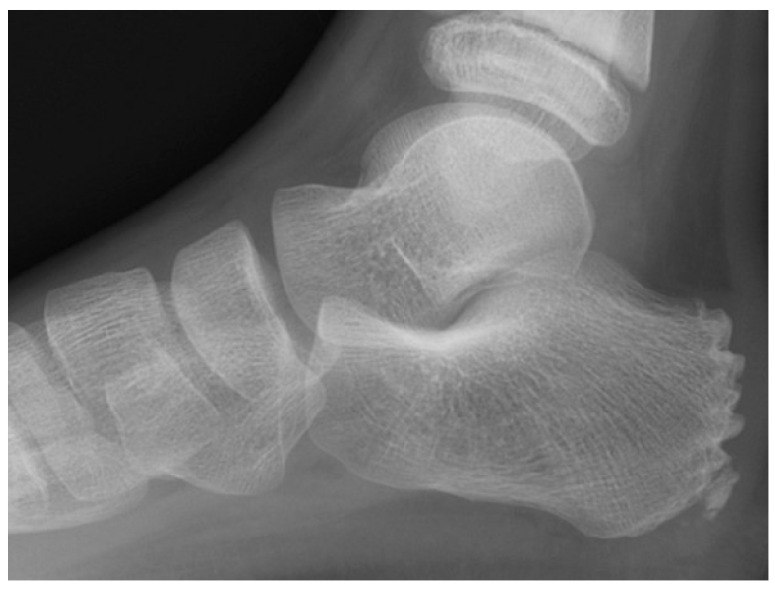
Radiograph of an 8-year-old asymptomatic male patient showing irregularity and fragmentation of the calcaneal apophysis.
